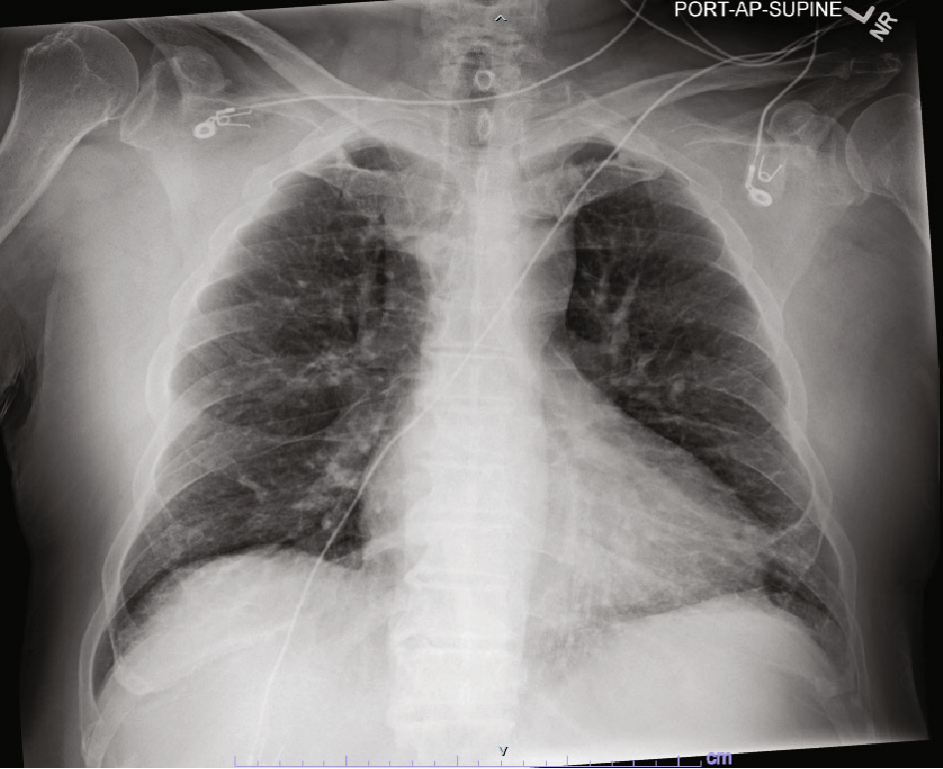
Chest x-ray demonstrating bilateral interstitial opacities concerning for pulmonary edema.
